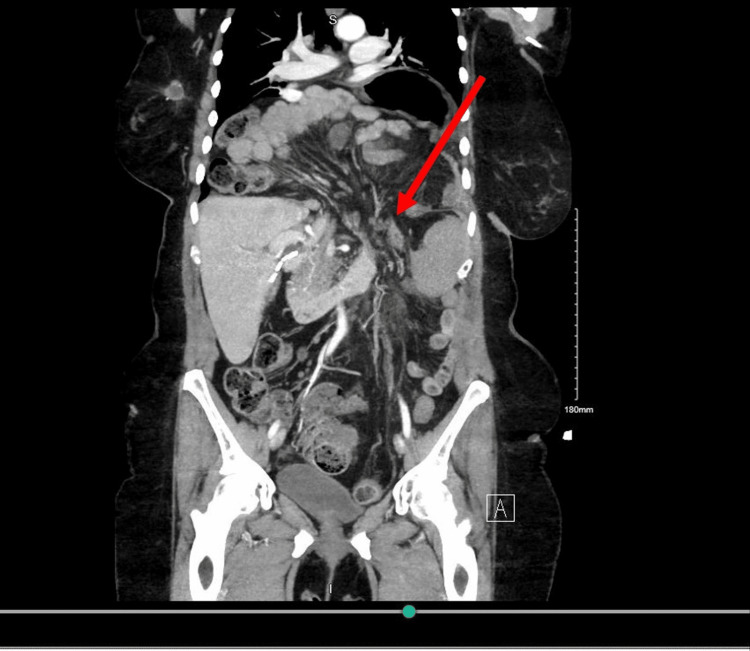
CT taken on readmission day 1 - image 1 The image demonstrated a recurrence of the hiatal hernia containing the majority of the stomach, large portions of small bowel, and transverse colon. Edema was imaged around the pancreatic tail, which was reflective of mild pancreatitis CT: computed tomography
